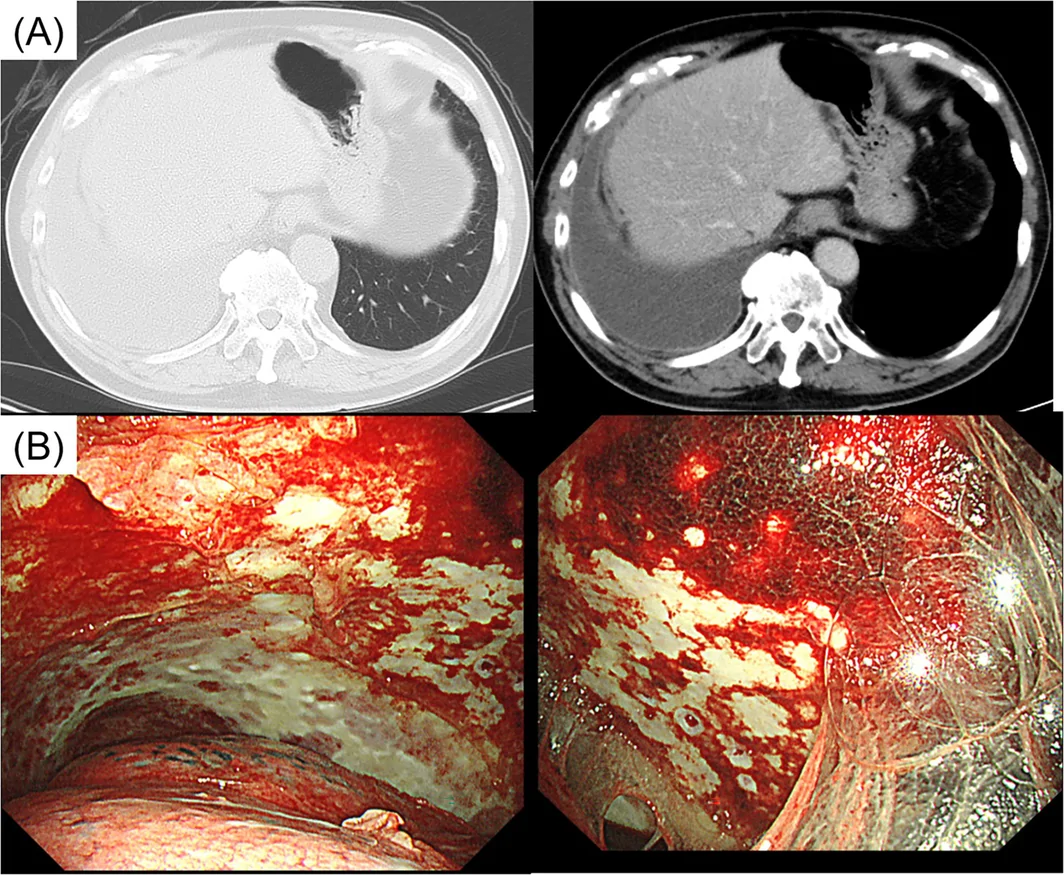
(A) Chest Computed Tomography (CT) at the initial presentation (November 2024) showing massive right pleural effusion. (B) Thoracoscopic view at the referring hospital showing purulent effusion and fibrin deposition in the pleural cavity.
